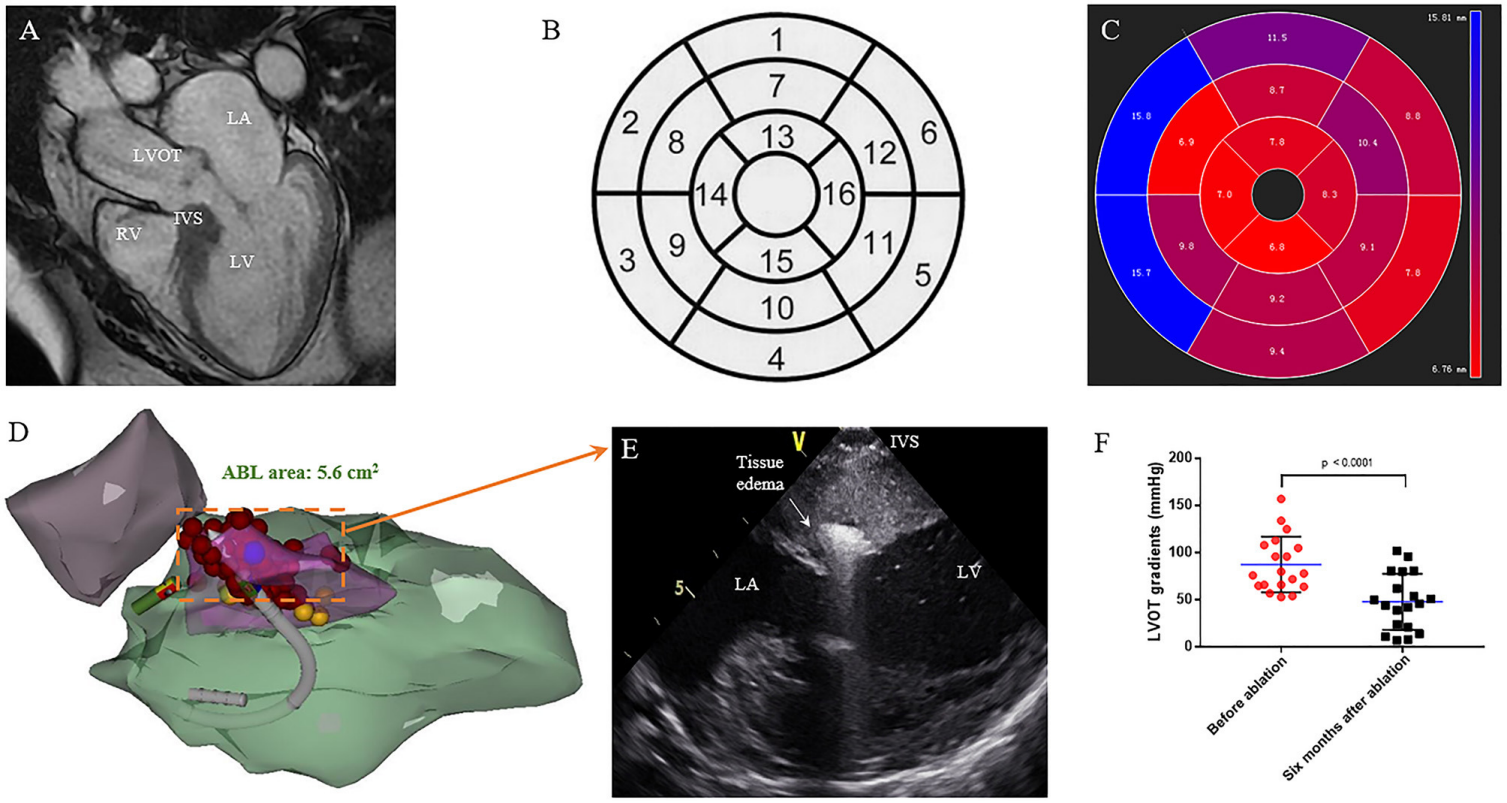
Interventricular septal ablation in patients with HOCM. (A) CMR end-diastolic long-axis image demonstrates a significant hypertrophy in basal septum. (B) Display of the 16 myocardial segments on a circumferential polar plot. The recommended nomenclature for tomographic imaging of the heart: 1. Basal anterior, 2. Basal anteroseptal, 3. Basal inferoseptal, 4. Basal inferior, 5. Basal inferolateral, 6. Basal anterolateral, 7. Mid anterior, 8. Mid anteroseptal, 9. Mid inferoseptal, 10. Mid inferior, 11. Mid inferolateral, 12. Mid anterolateral, 13. Apical anterior, 14. Apical septal, 15. Apical inferior, 16. Apical lateral. (C) The circumferential polar plot presents a limited hypertrophy in basal anteroseptal and inferoseptal segments. The number indicates the average thickness of each segment. (D) Right anterior oblique (RAO) projection of the CARTO shell. Left ventricular (green), aorta (purple), SAM area of hypertrophic septum (pink), and left bundle branch (yellow) are presented. Ablation is performed on SAM area (red). (E) On the live ICE screen, significant tissue edema can be observed after ablation. (F) Reduction of obstruction at rest is seen on continuous-wave Doppler echocardiography. The LVOT gradient (in mmHg) is significantly decreased after 6 months follow up. (*P* = 0.001) LA, left atrium; LV, left ventricle; LVOT, left ventricular outflow tract; RV, right ventricle; IVS, interventricular septum.
